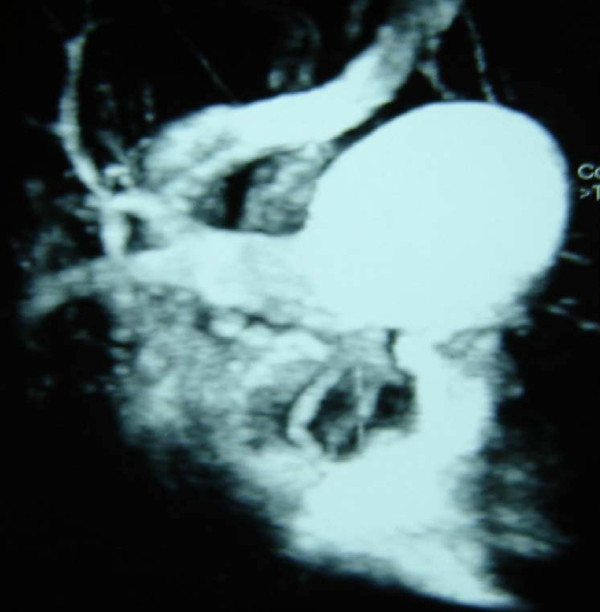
Chest spiral CT angiography of the first patient (Case 1) showing the aneurysmal dilation involving the pulmonary trunk and its bifurcation.
